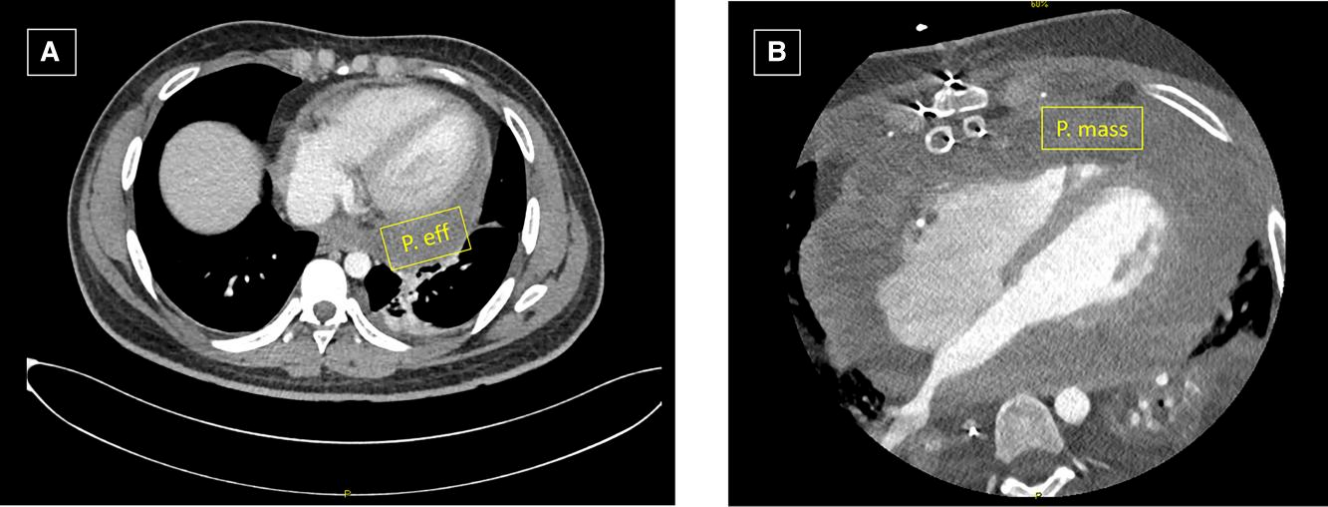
Chest computed tomography showing pericardial thickening with moderate pericardial effusion (P. eff) (*A*) and large pericardial mass (moreover) with compression of left atrium (LA) 4 months later (*B*).
